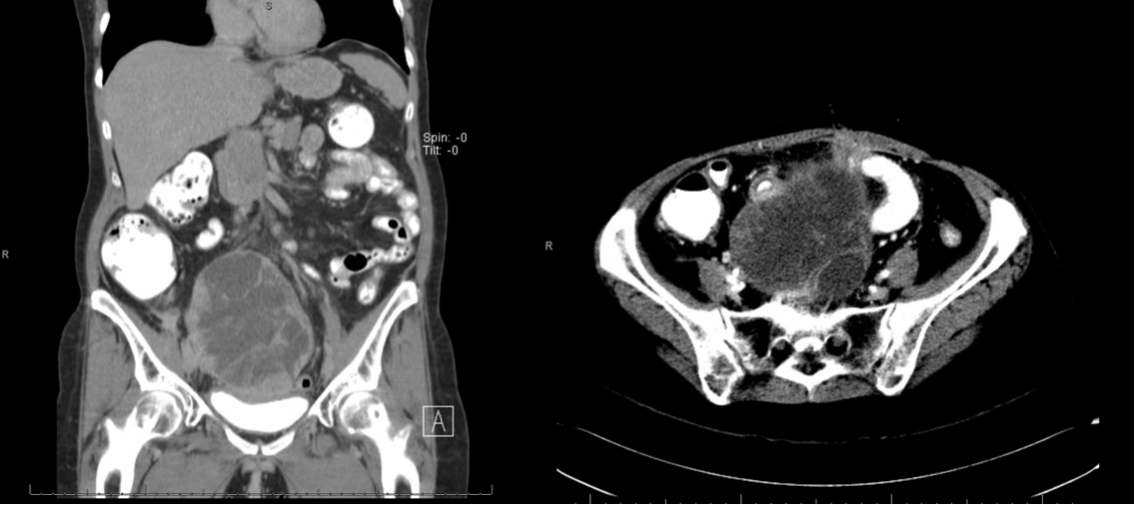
Abdominal CT scan at first follow-up Abdominal CT shows interval decrease in size of the large heterogeneous pelvic mass 4 months after radiation therapy. (February 2014)
